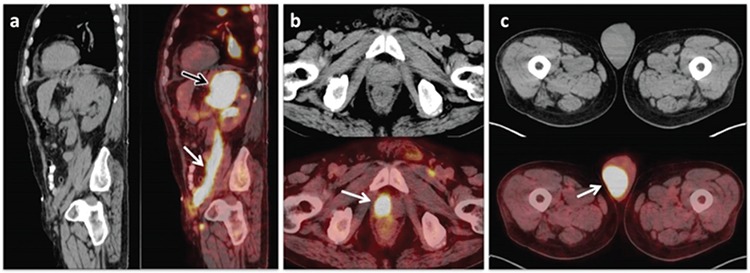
At sagittal CT and fused PET/CT images (A); mass lesion at the left adrenal gland (A; black arrow) and intense ^18^F-FDG uptake (SUV_max_: 36.8) along the left testicular vein (A; white arrow) are observed. At transaxial CT and fused PET/CT images (B, C); diffuse increased ^18^F-FDG uptake without size or density changes at CT image suggesting diffuse lymphomatous infiltration on prostate (SUV_max_: 40.4) (B) and right testicle (SUV_max_: 20.8) (C) are also seen.
